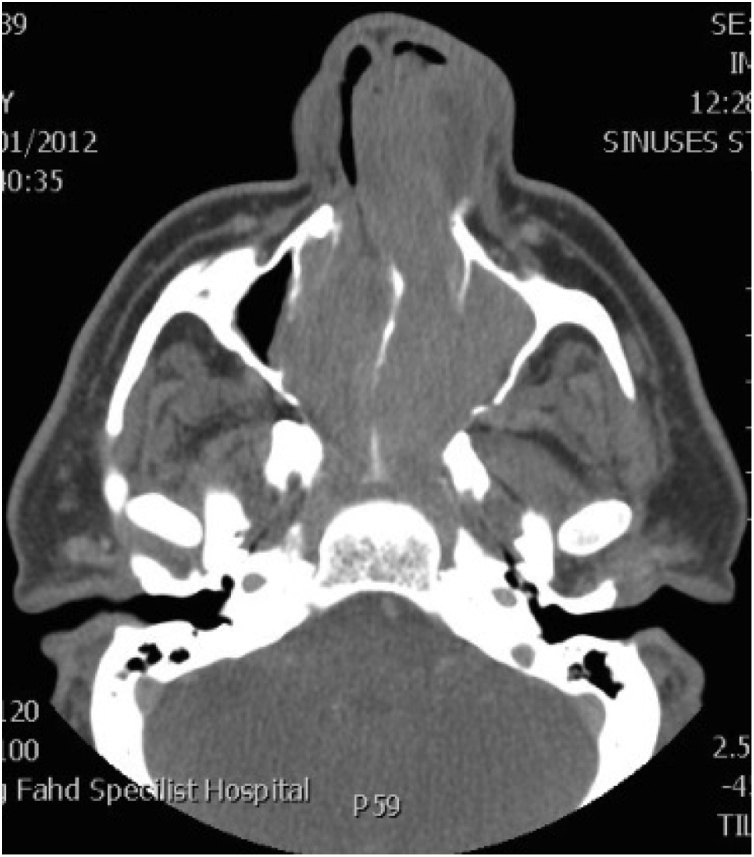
CT scan of the para-nasal sinuses showed bilateral homogenous opacity filling both nasal cavities and the left maxillary and frontal sinuses.
